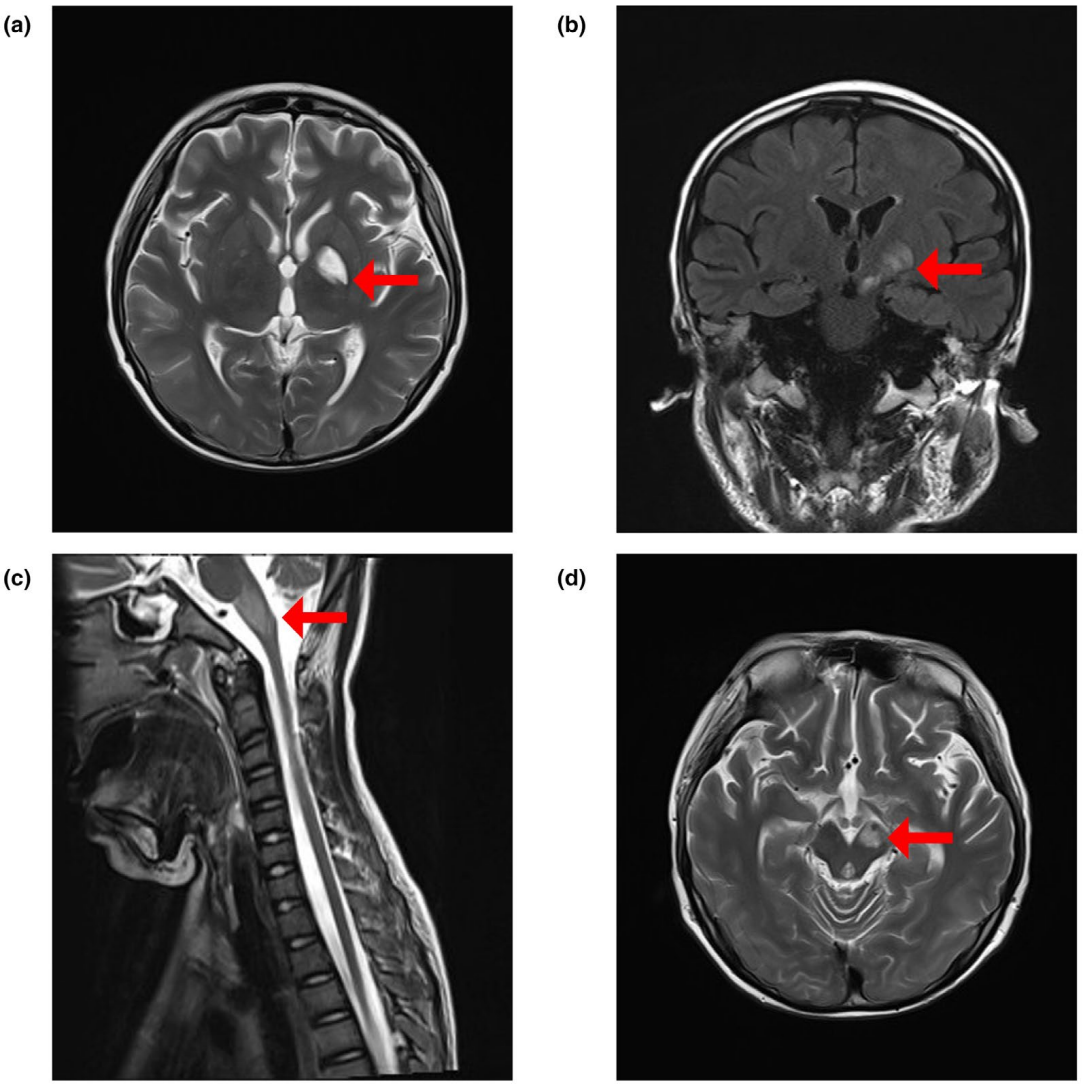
MRI of the head and spinal cord showing multiple lesions. (a and b) Axial T2‐weighted and coronal FLAIR brain MRI shows hyperintensity in the left basal ganglia region (red arrow). (c) Sagittal T2‐weighted cervical spine MRI shows hyperintensity in the medulla oblongata (red arrow). (d) Axial T2‐weighted brain MRI shows hyperintensity in the left midbrain (red arrow)
